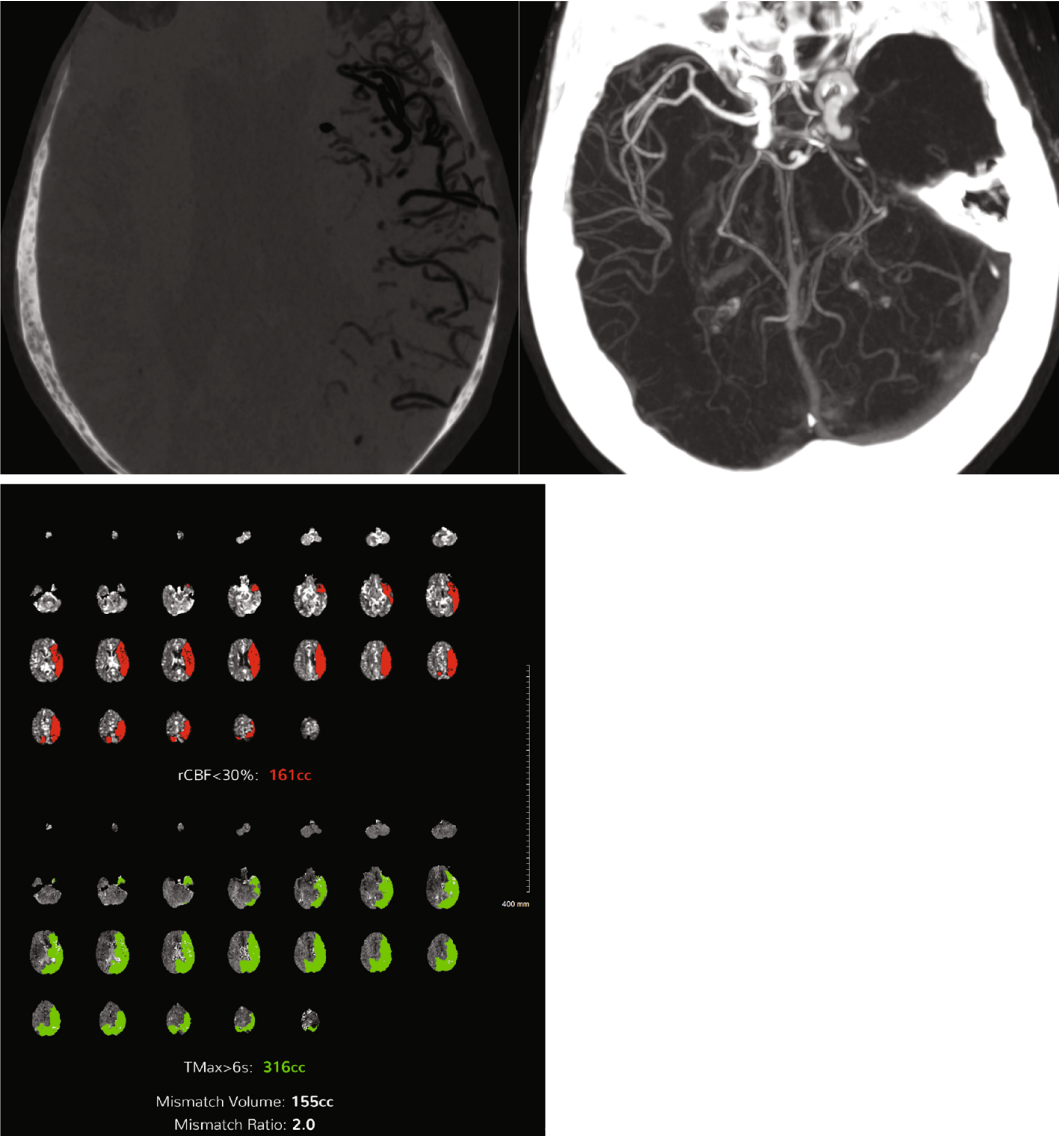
Maximal intensity image (MIP) and minimal intensity image (MIN) demonstrate complete occlusion of the left MCA vessels. CT brain perfusion software analysis demonstrates a large area of mismatch volume of 155 cc in the left MCA distribution.
